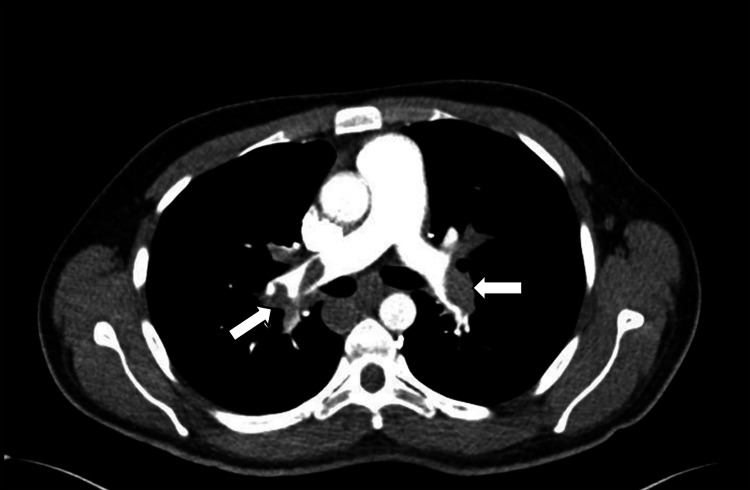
Axial view of CTPA in the arterial phase showing filling defects (acute PE) involving the left and right main pulmonary arteries (arrows). CTPA: computed tomography pulmonary angiogram, PE: pulmonary embolism.
